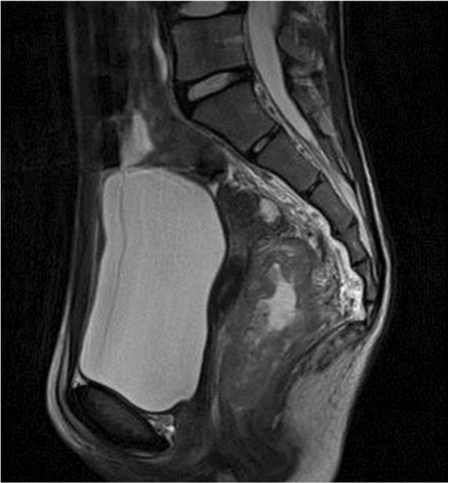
MRI of sagittal plane revealed a balky tumor in lower rectum, measured 76 mm in diameter
